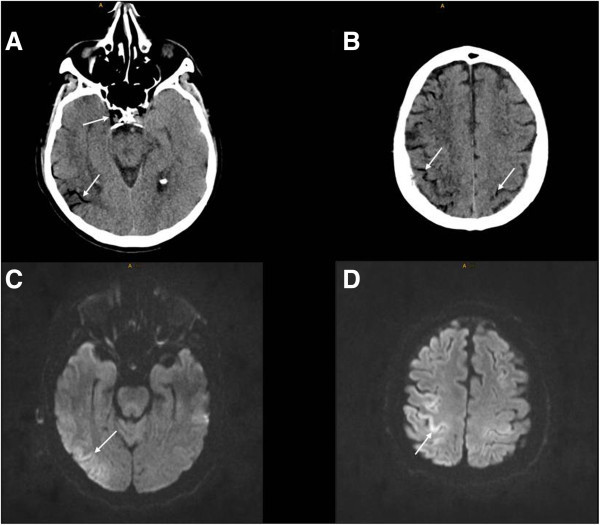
CT and MRI in cerebral air embolism. The initial head CT shows air entrapment in cortical branches of both middle cerebral arteries and in the cavernous sinus (arrows in A and B). On day ten MRI of the brain demonstrates cortically localized areas of restricted diffusion along the gyri (arrows in C and D).
